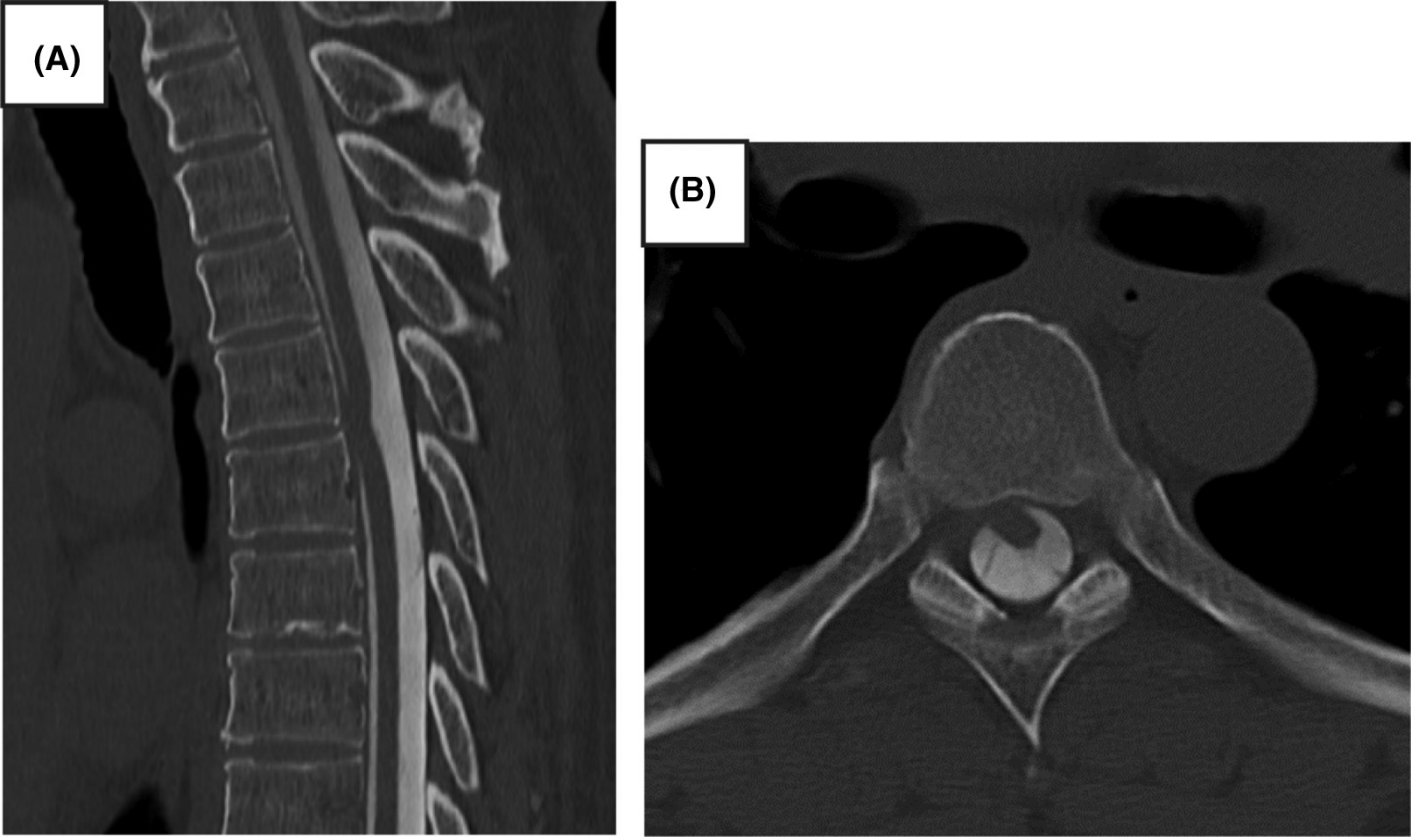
Preoperative computed tomography (CT) myelogram. (A) Sagittal CT myelography showing anterior deviation and flexion of the spinal cord at the Th6/7 level. (B) Axial CT myelogram showing ventral deviation of the spinal cord at the Th6/7 level.
